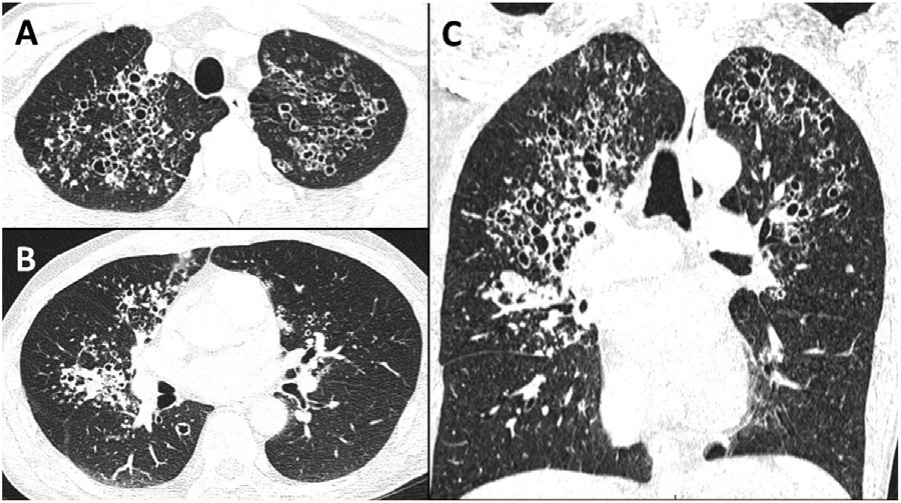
Axial CT image at the apex (A) and at the level below the tracheal bifurcation (B). Coronal CT image of the lungs (C).
